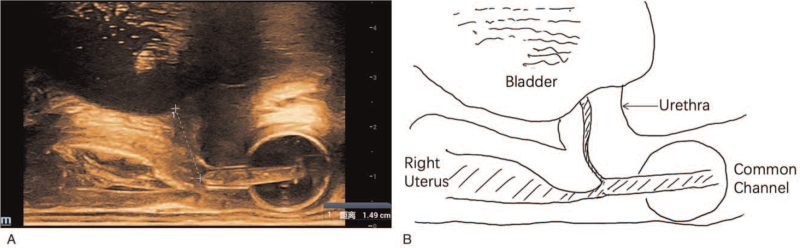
Contrast-enhanced 2-dimensional transrectal biplanar high-frequency ultrasonography showing the confluence of urethra and vagina.
